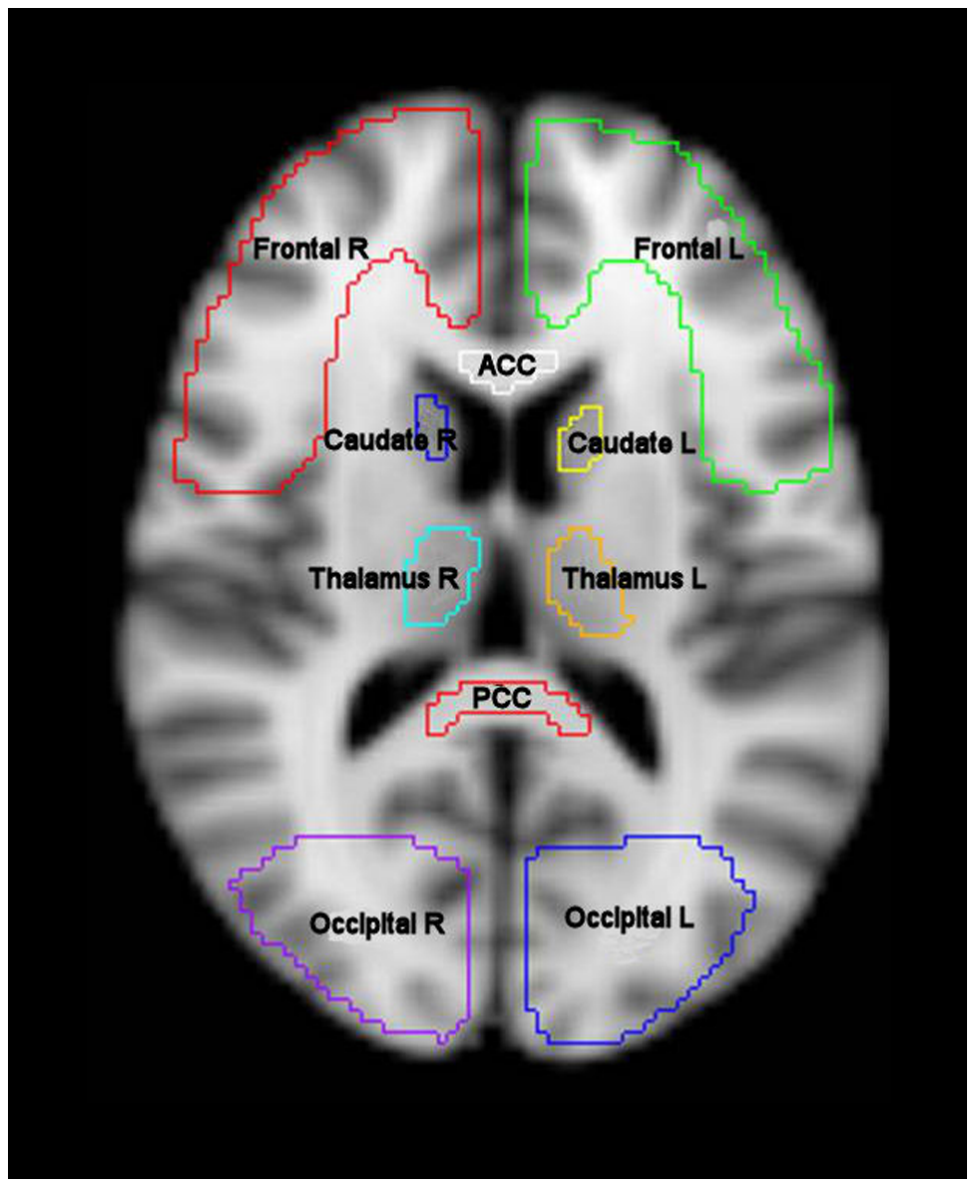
Region of interest template. T1 weighted magnetic resonance image in MNI152 space (2 mm resolution) showing frontal lobe left (Frontal L), frontal lobe right (Frontal R), anterior corpus callosum (ACC), caudate left (Caudate L), caudate right (Caudate R), thalamus left (Thalamus L), thalamus right (Thalamus R), posterior corpus callosum (PCC), occipital left (Occipital L) and occipital right (Occipital R). Additional regions not shown include body corpus callosum, ventral midbrain, dorsal midbrain, forceps minor, forceps major and bilateral regions covering the hippocampus, parietal lobe, temporal lobe, cerebral peduncle, pons, cerebellum, anterior thalamic radiation, superior longitudinal fasciculus, inferior longitudinal fasciculus, cingulum, uncinate fasciculus and corticospinal tract.
